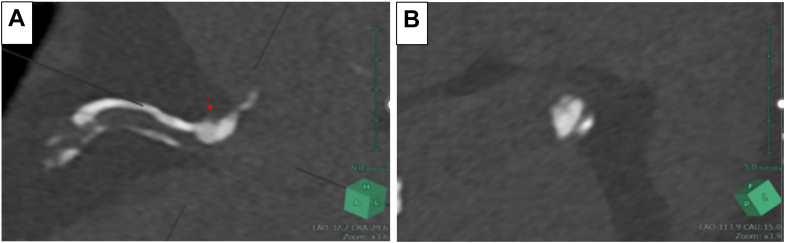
Thin-Slice Computed Tomography Image (A) The arrow indicates the thick calcified lesion at the ceiling of the right coronary artery ostium. (B) A short-axis view of the lesion shows a slit-like lumen wedged between calcified plaques.
